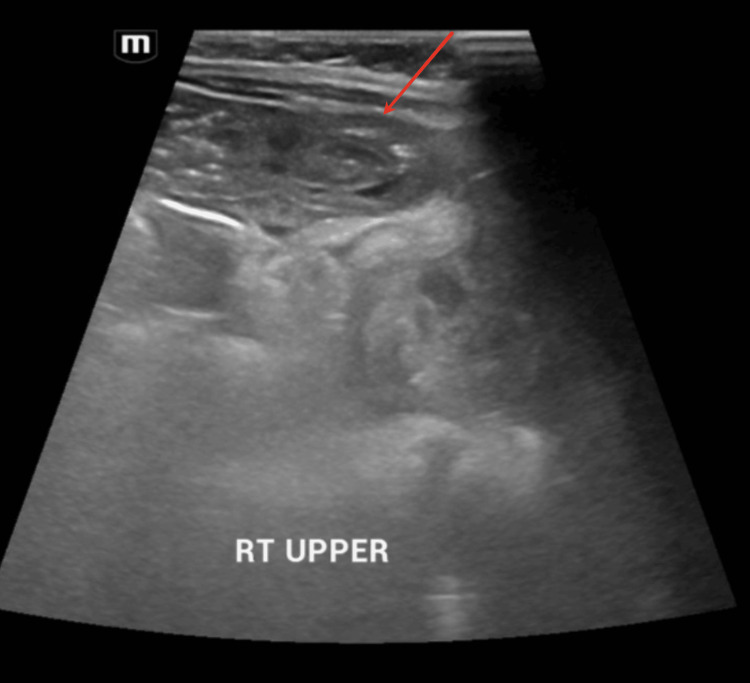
Ultrasound abdomen longitudinal view showing a pseudokidney appearance Red arrow pointing at pseudokidney appearance
